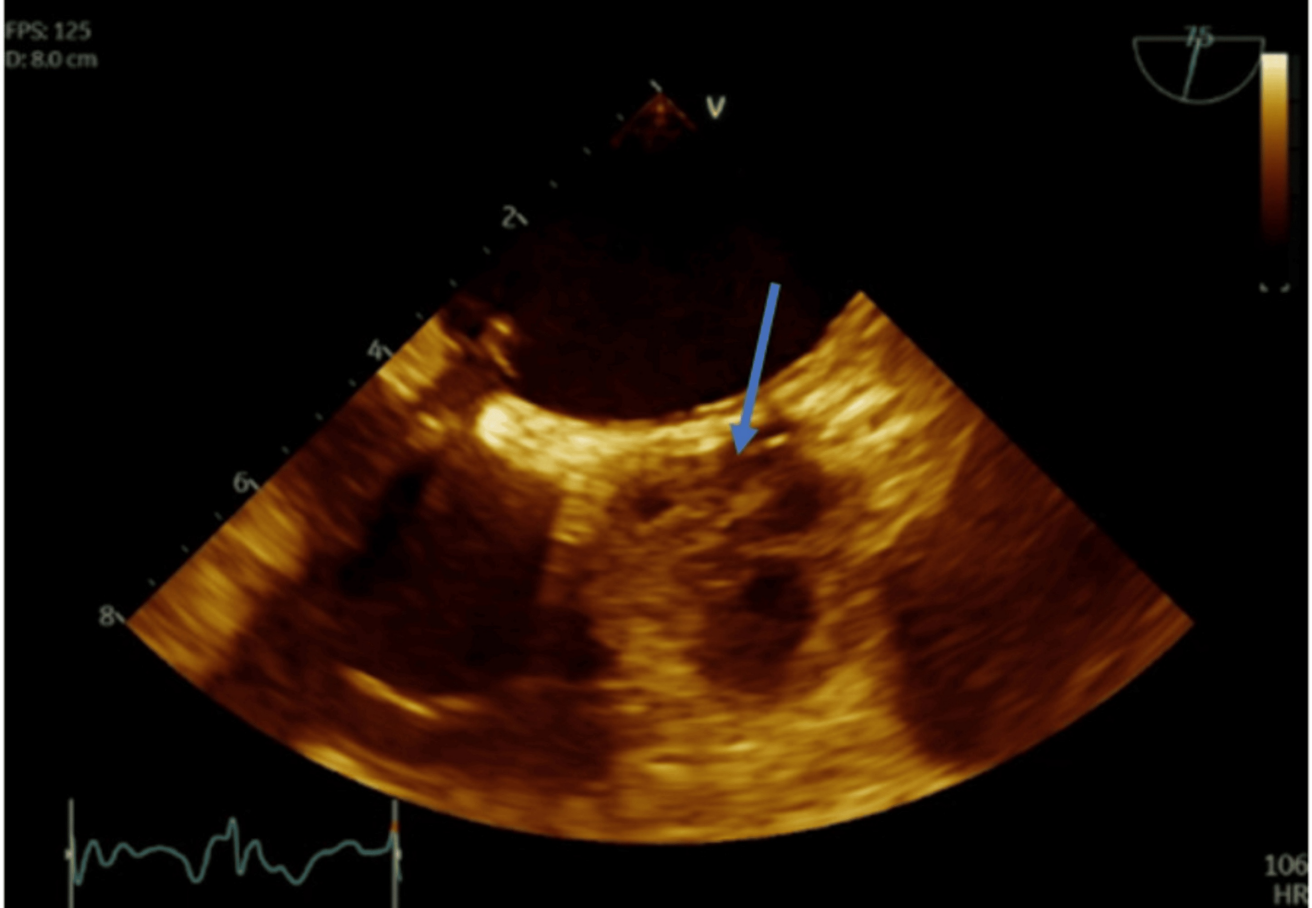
TEE, mid-esophageal short-axis view, zoomed out, showing thrombus of the AV (arrow) TEE: Transesophageal echocardiogram; AV: Aortic valve
